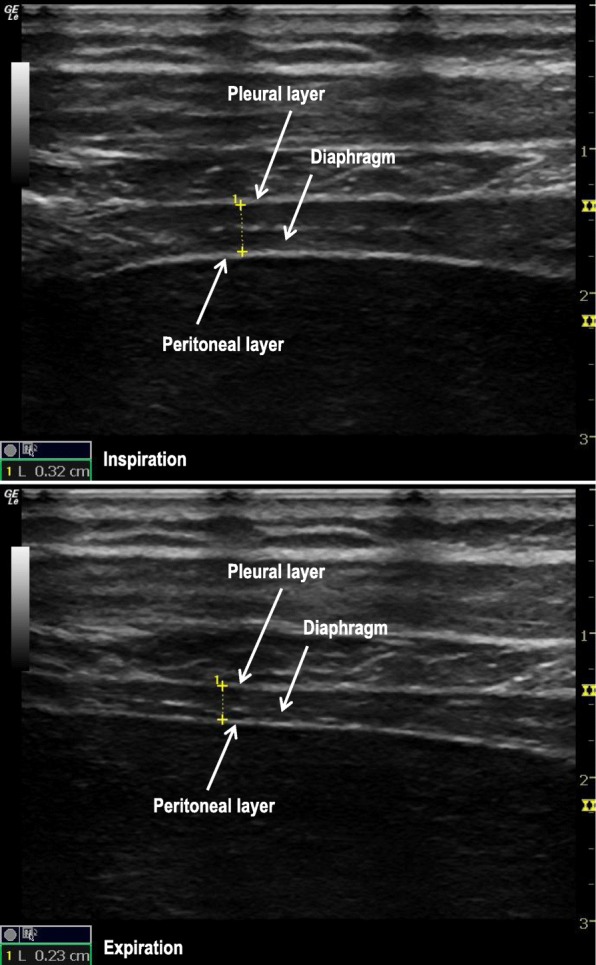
Ultrasound assessment of diaphragmatic thickness and diaphragmatic thickening fraction (DTF). Diaphragmatic thickness was assessed in B-mode at end-inspiration (upper panel) and end-expiration (lower panel) during quiet breathing. The white arrows indicate pleural layer and peritoneal layer. Diaphragm is between the 2 layers. The dashed line between the 2 crosses indicates diaphragm thickness; its value is shown at left lower corner of the image. DTF is defined as the diaphragmatic thickness at end-inspiration minus the diaphragmatic thickness at end-expiration (TEE) divided by the TEE, expressed as percentage
